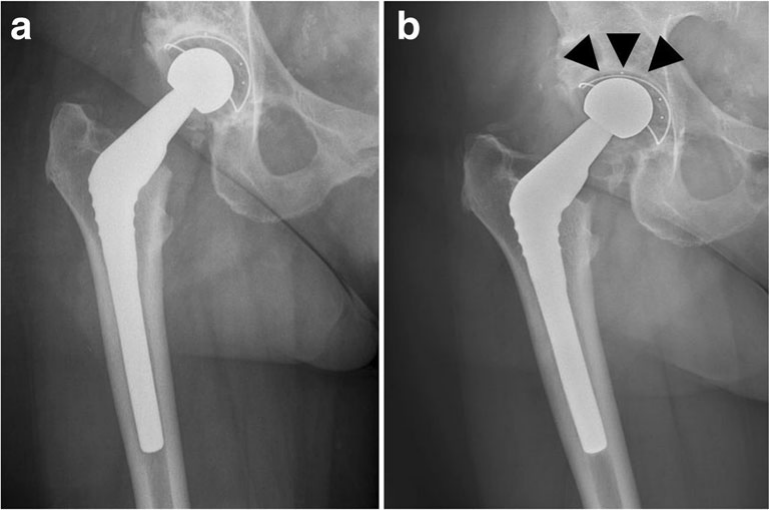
a AP radiograph, 6 months postoperatively. Cementless total hip arthroplasty. Normal postoperative findings. b AP radiograph of the same patient, 4 years postoperatively. Cranial displacement of the femoral head in the acetabular cup (*arrowheads*), indicating linear wear
